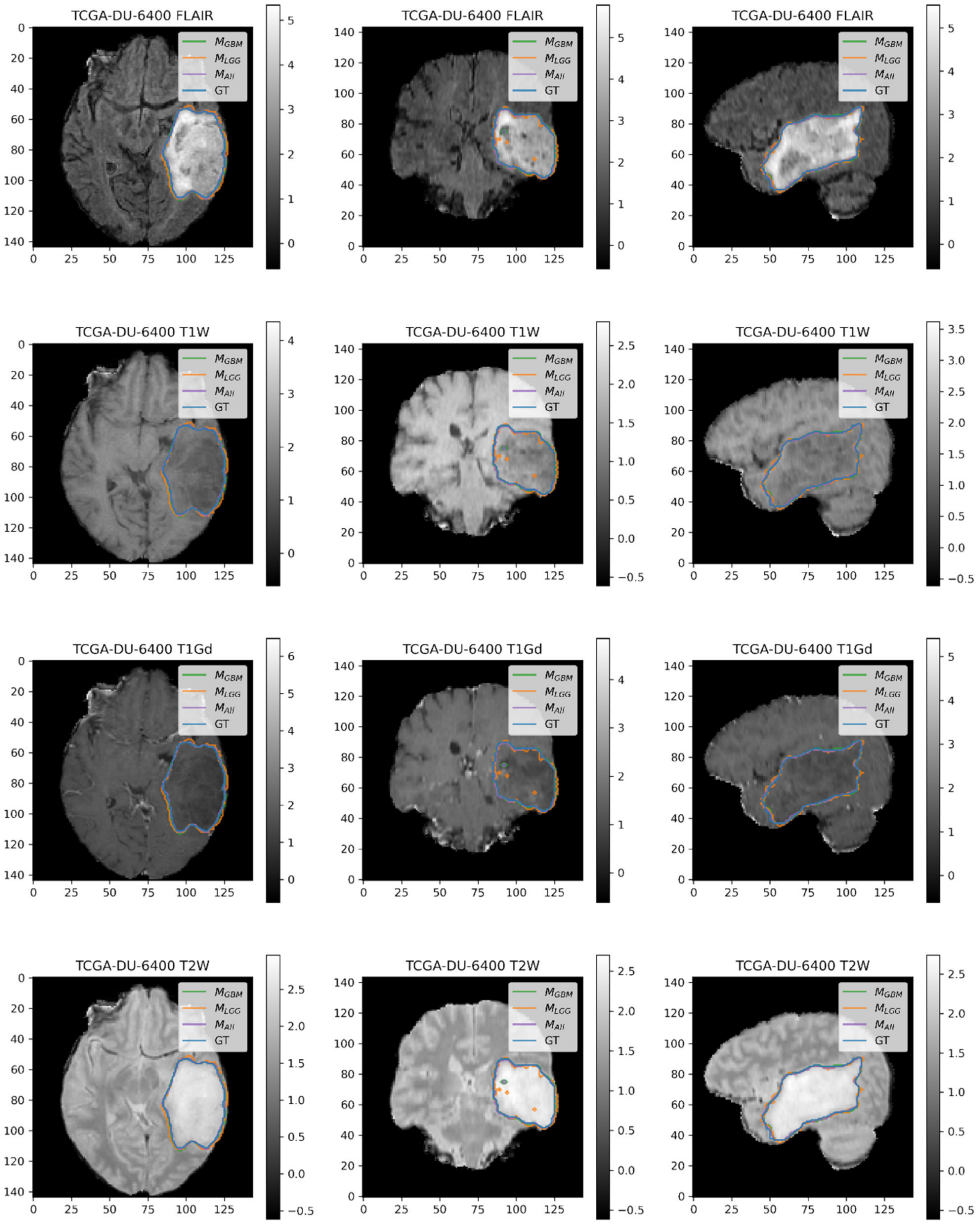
Patient TCGA-DU-6400 belongs to *D*_*LGG*_ and has a tumor in the left temporal parietal region. We selected this patient as an example because it has consistently good performance for metrics (Sens, B.Acc, Dice, Jac.C) across all models. This LGG has clear margins, and the classic signature of FLAIR enhancement and no T1-Gd enhancement.
